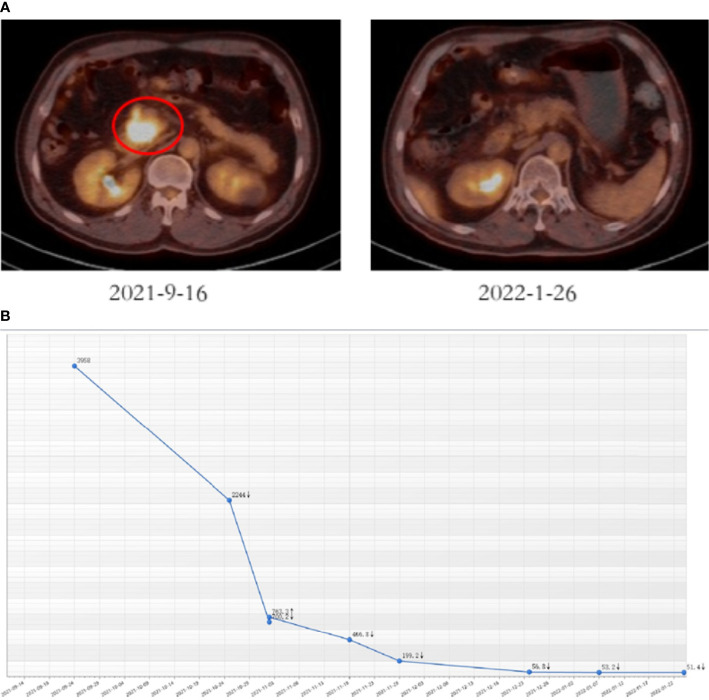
Tumor responses after induction therapy. (A) PET-CT was performed before (size: 4.1 cm * 3.6 cm, SUV = 18.2) and after 4 cycles of the treatment (size: 2.3 cm * 1.5 cm, SUV = 4.8). (B) CA19-9 levels of this patient during the treatment.
